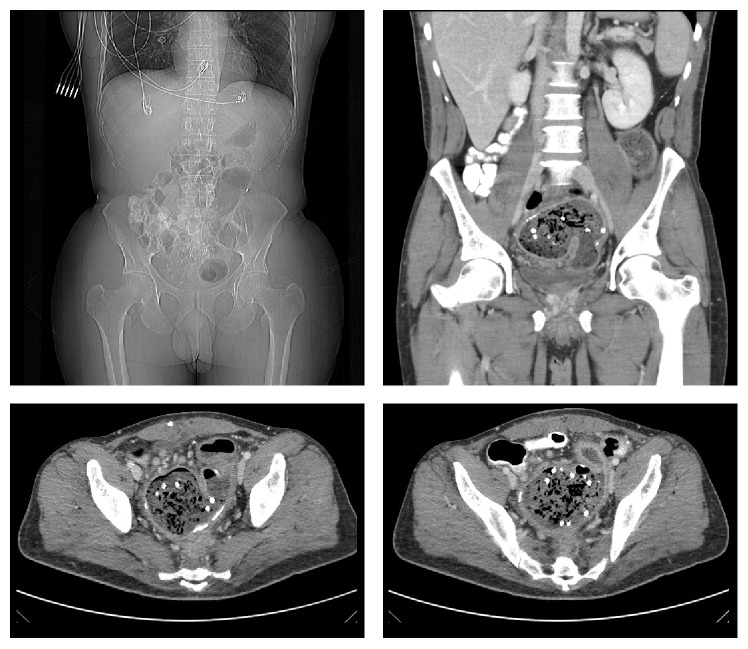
CT scan showing thickening and stranding of the J-pouch and rectum, with obliteration of the rectal fat planes, along with mesenteric lymphadenopathy. There was proximal dilatation with partial air-fluid levels within the small bowel, concerning for partial obstruction.
